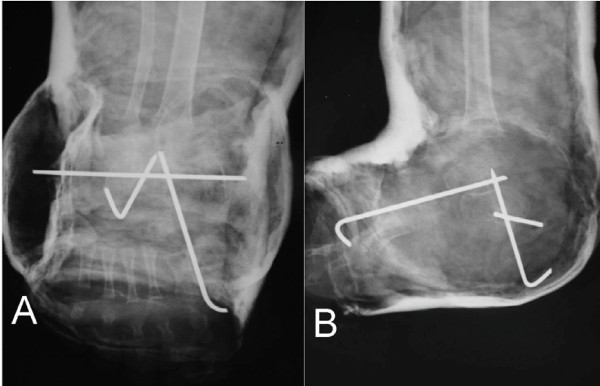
A; AP post-operative X-ray, showing convergence between the talus and calcaneus (33° Kites angle), and AP positive(5°) talus-1^st ^MT angle, B; lateral view showing immediate correction of the lateral talo-calcaneal angle (36°), and an obtuse tibio-calcaneal angle.
